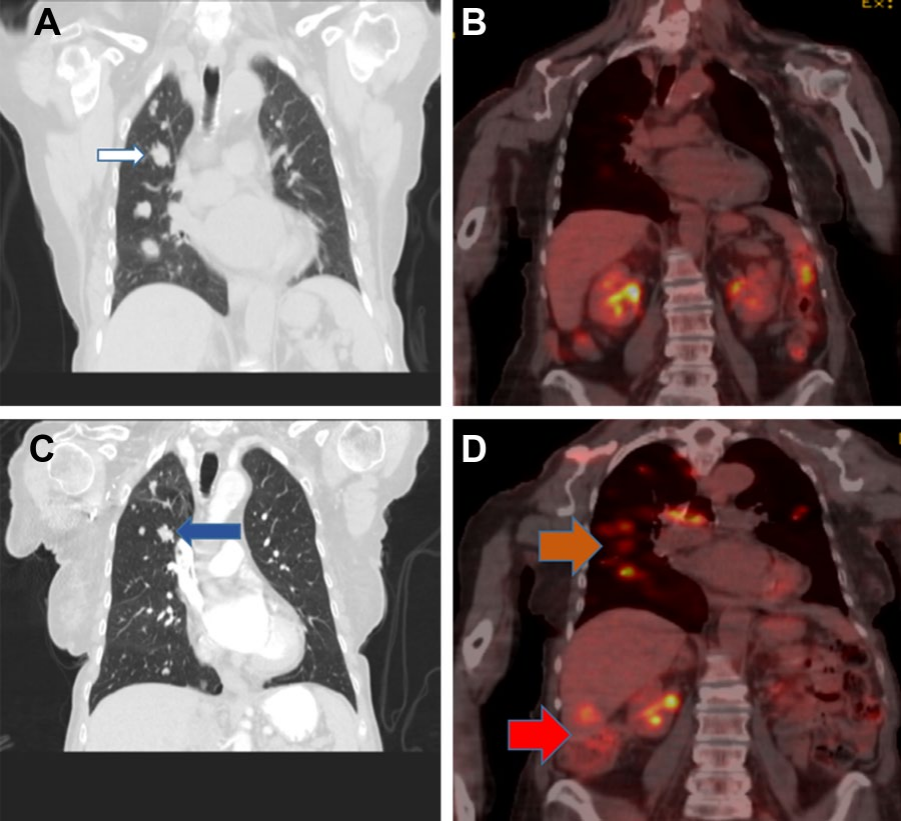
(A) CT chest shows pulmonary metastasis (white arrow). (B) PET-CT shows very good partial response. (C) CT chest shows pulmonary disease progression (blue arrow). (D) PET-CT scan shows pulmonary and hepatic disease progression (orange and red arrows, respectively).
